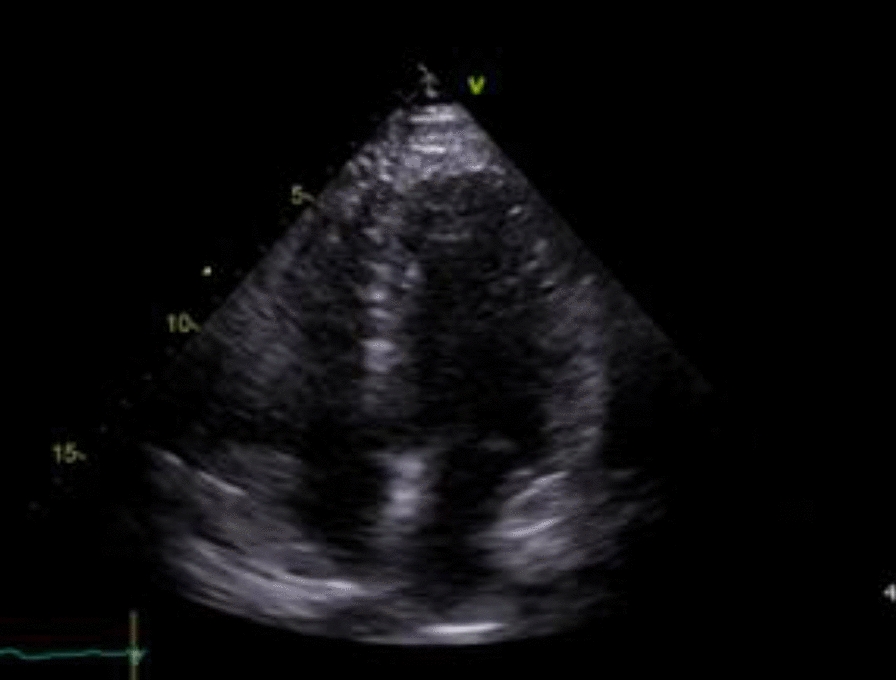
Post-procedural thoracocentesis transthoracic echocardiogram showed the resolution of tamponade
